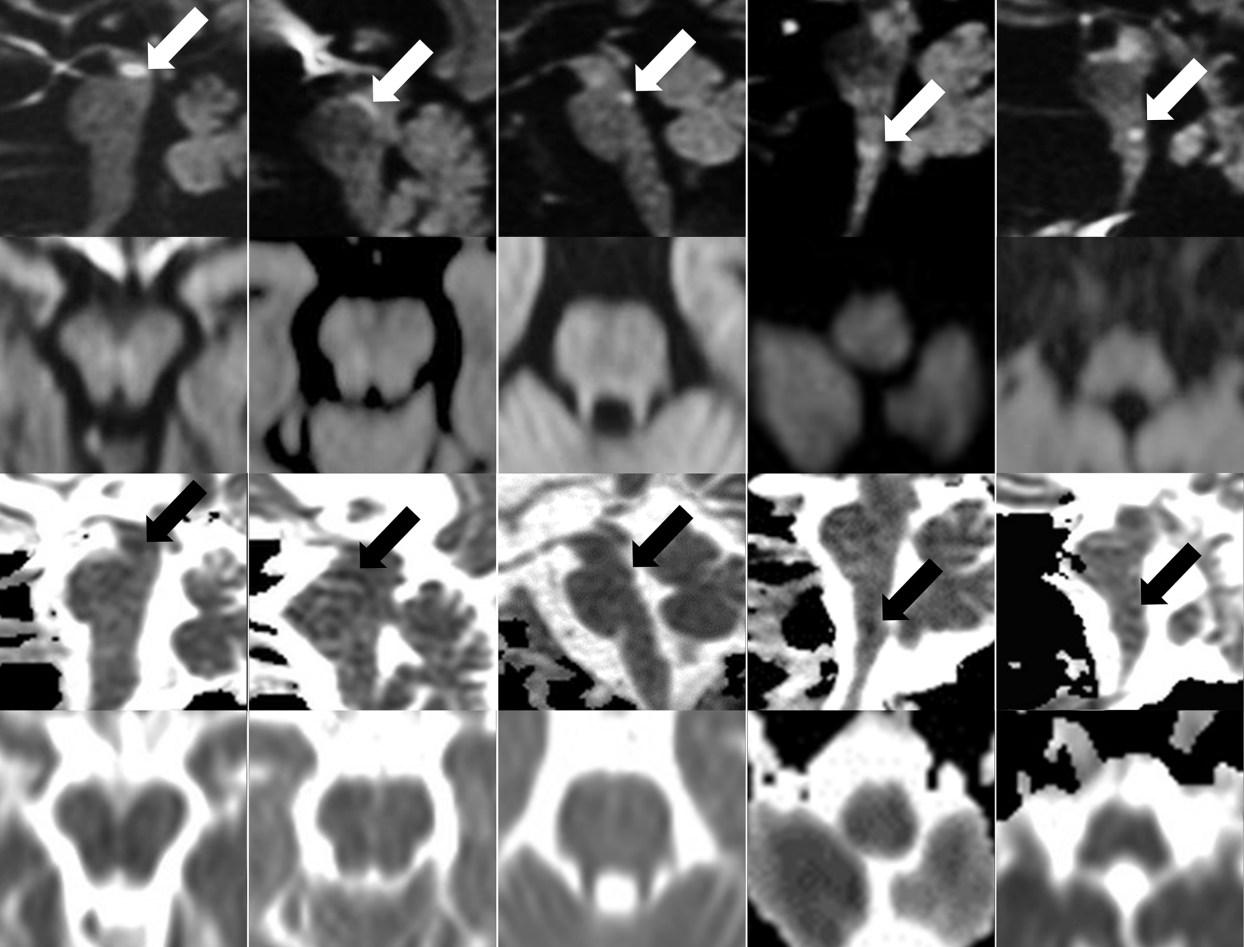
Lesions additionally detectable on sagittal DWI. Sagittal (first row) and corresponding axial (second row) B-1000 images and ADC-maps (third and fourth row) of the five DWI lesions (arrows) that were additionally detectable on the sagittal DWI (R_SAG).
